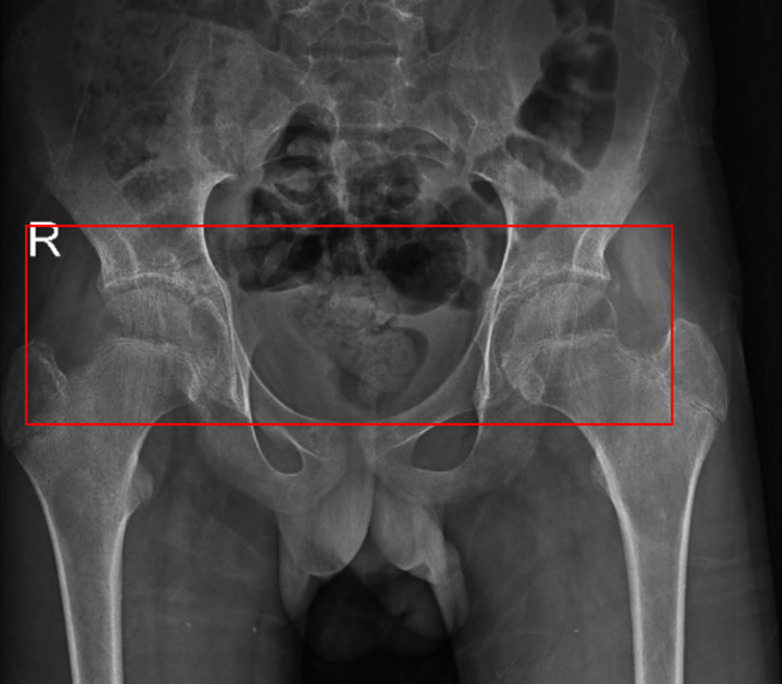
magnetic resonance imaging scan indicated bilateral hip arthritis with bilateral sacroiliitis, T2/PDFS hyperintensity was noted in the bilateral sacroiliac joint, erosion of bilateral acetabular surface, minimal bilateral hip joint effusion with marginal erosion along the articular surface, T2/PDFS hyperintensity was noted in the neck of the femur and greater trochanter of bilateral femur S/O edema
